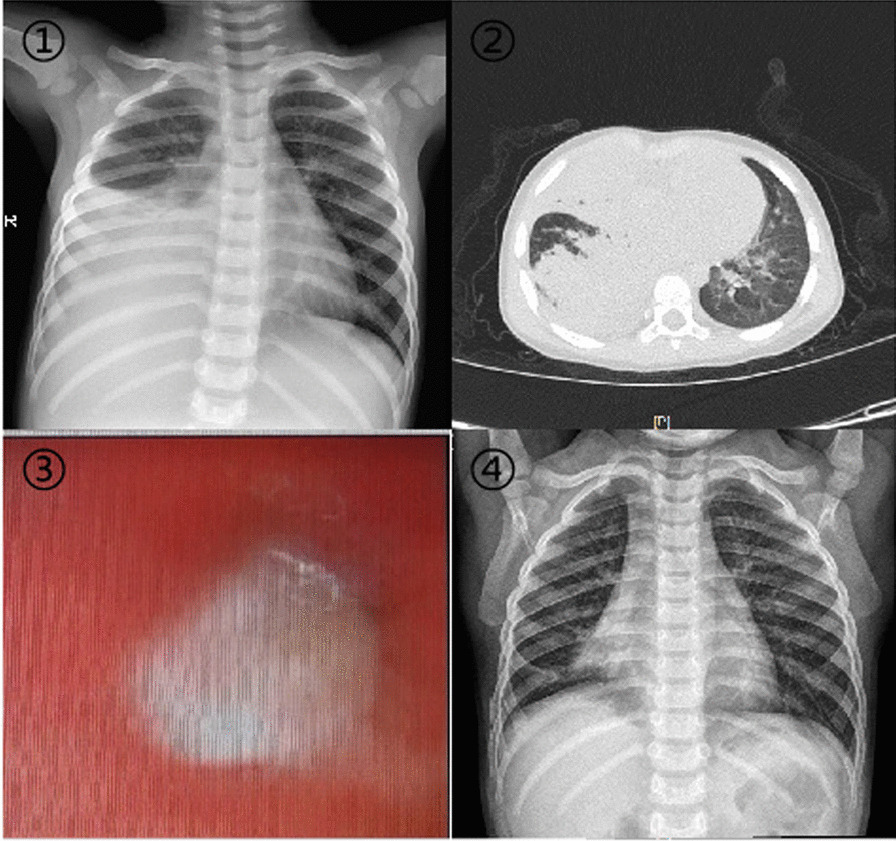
A case of plastic bronchitis caused by adenovirus infection. 1: Chest radiograph on admission: Pulmonary consolidation and atelectasis in the middle and lower lobes of the right lung. 2: Lung computed tomography on the second day after admission: Pulmonary consolidation and atelectasis in the middle and lower lobes of the right lung. 3: An obstructed lung lobe under bronchoscopy. 4: Chest radiograph on discharge: The pulmonary consolidation and atelectasis are resorbed
